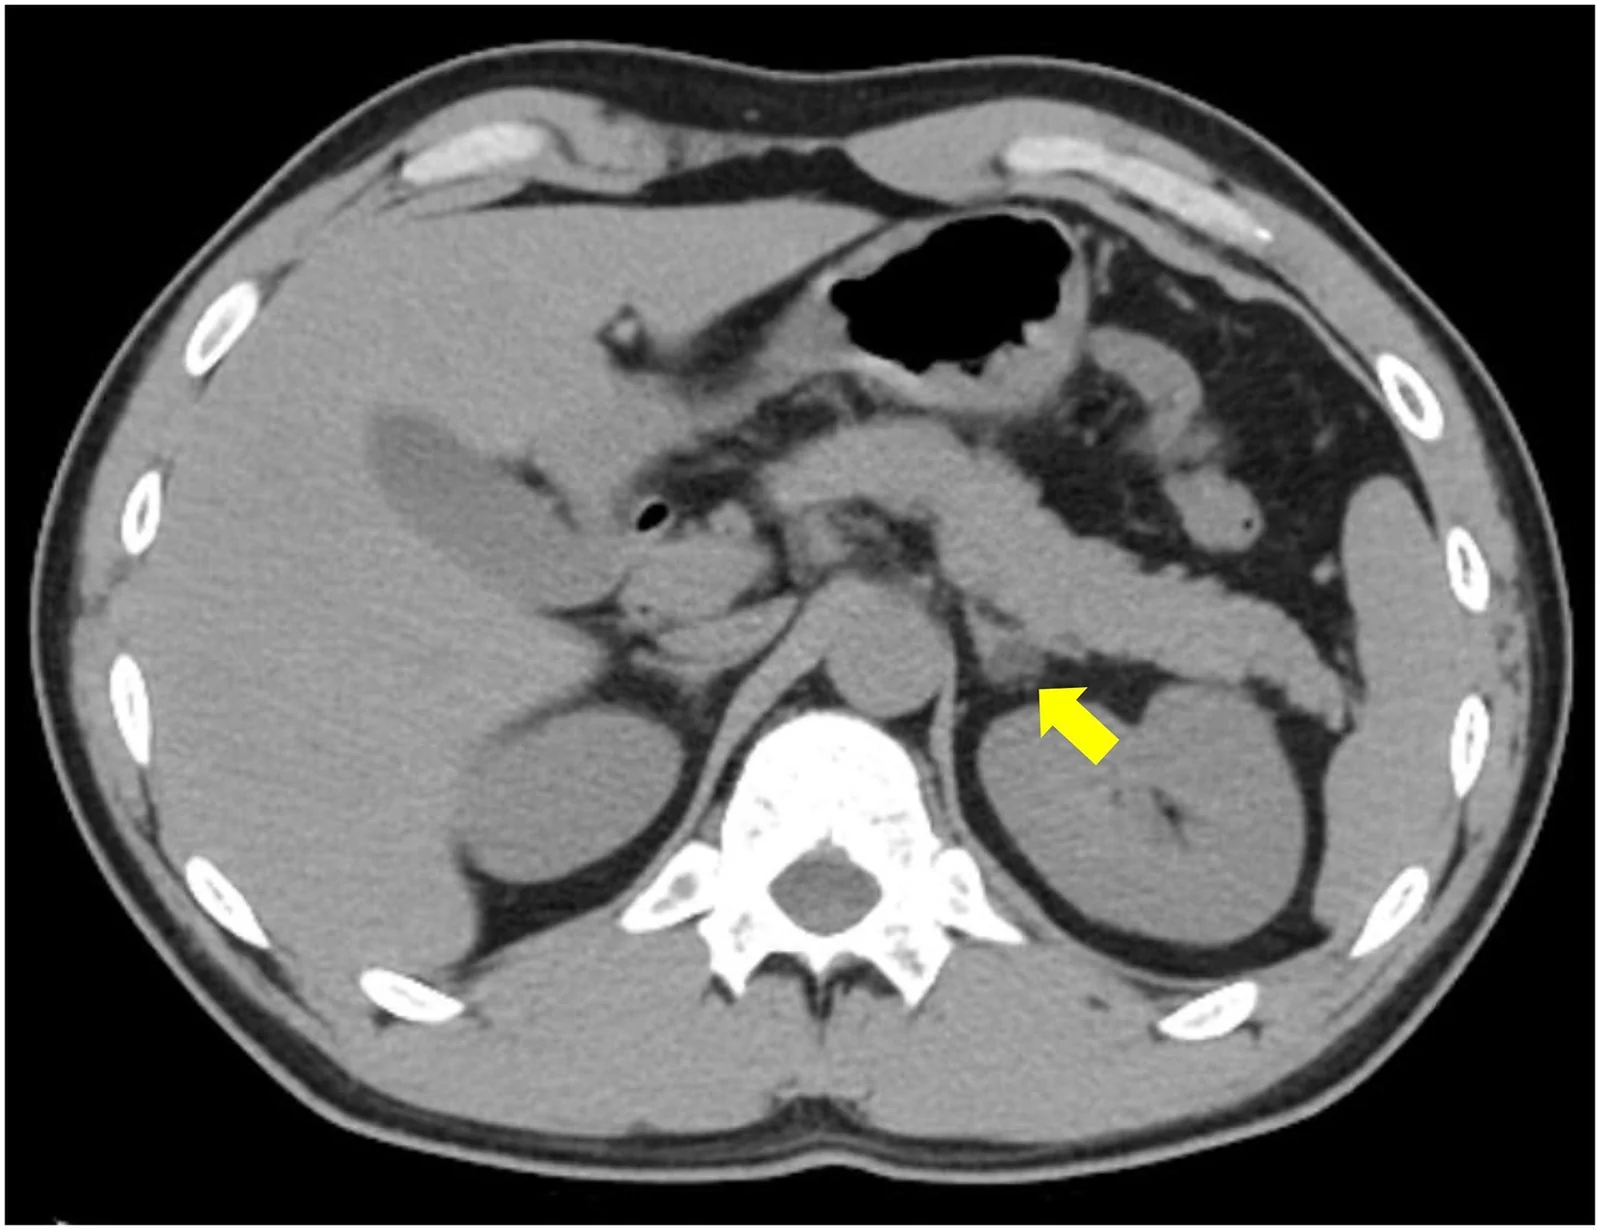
Abdominal CT scan. An unenhanced abdominal CT scan showing a 1.1-cm left adrenal tumor (arrow) with an attenuation value of −10 Hounsfield units.
